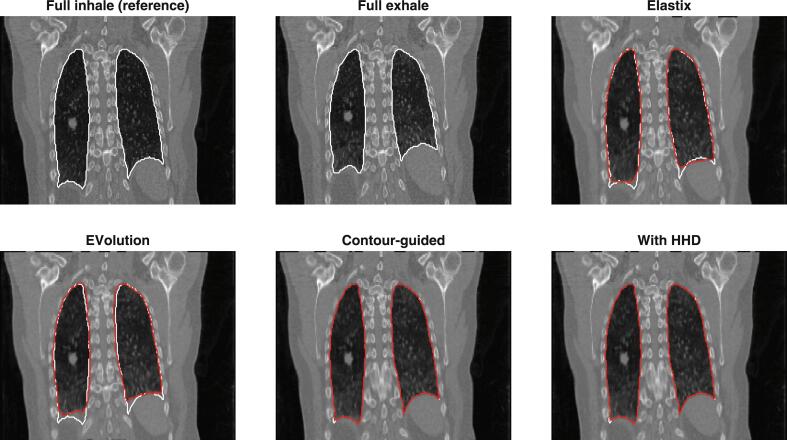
An example case for the experiment on large complex deformations of the thorax with CT-to-CT registrations. A coronal slice of the full inhale and full exhale images is shown (TRE before registration 10.9 mm), as well as the exhale image registered to the inhale using Elastix (TRE 2.9 mm), the original EVolution (3.9), our proposed contour-guided algorithm (1.7), and this contour-guided algorithm with the Helmholtz-Hodge decomposition (HHD) on the body excluding the lungs (1.8). The lung contours used for guidance are shown in white and the registered contours are shown in red. In particular, the caudal side of the lungs is better aligned when using contour-guidance.
